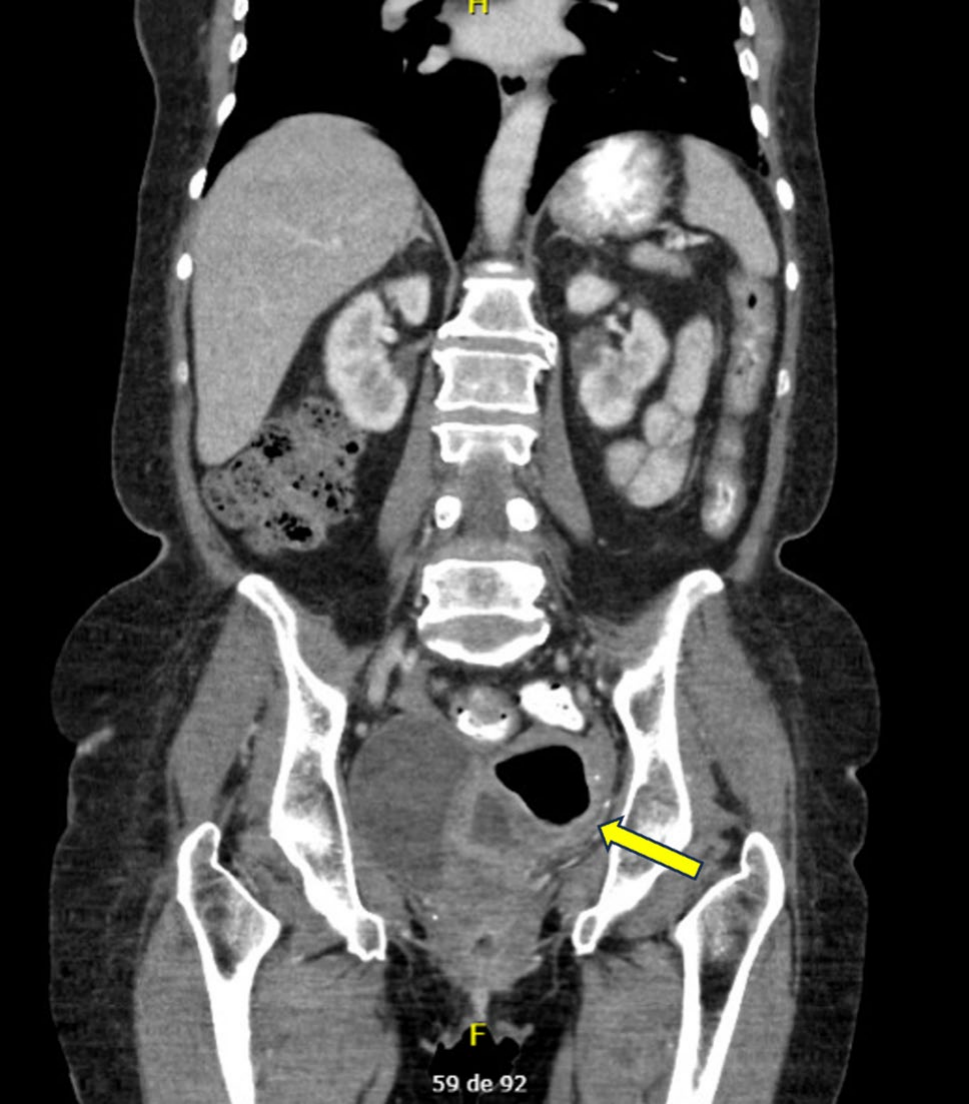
Coronal CT scan showing an air-fluid level within the uterine cavity
